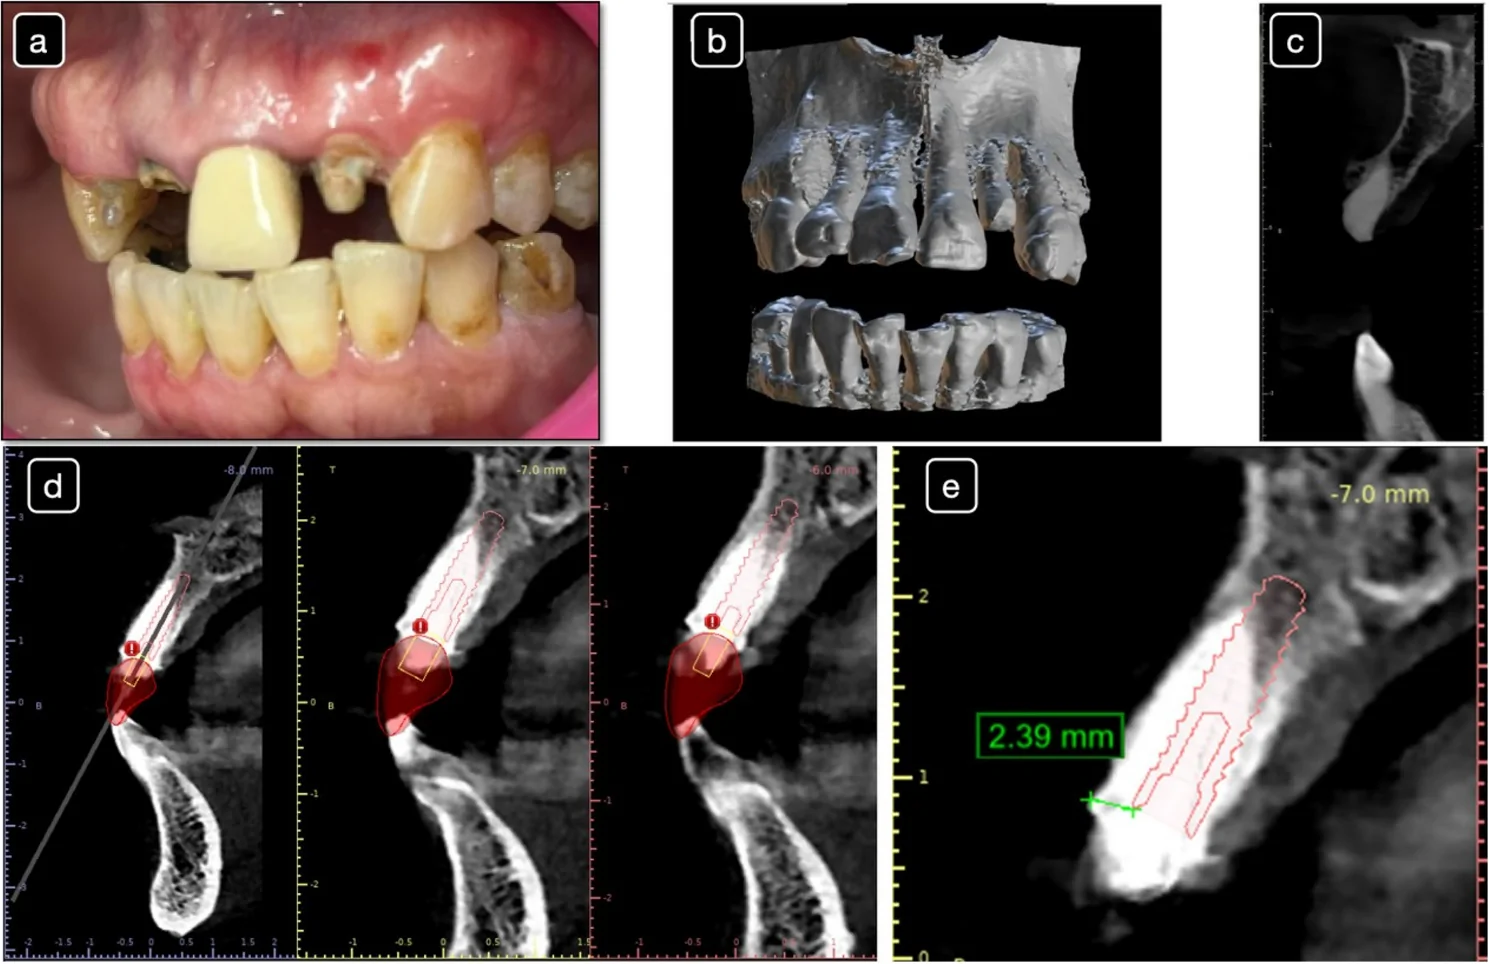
Preoperative assessment and virtual planning. a Preoperative clinical photograph. b Three-dimensional CBCT reconstruction. c Sagittal CBCT view of the site of interest confirming the absence of periapical pathosis. d Prosthetically driven virtual planning of the implant in its ideal position. e Virtual measurement of the jumping gap
